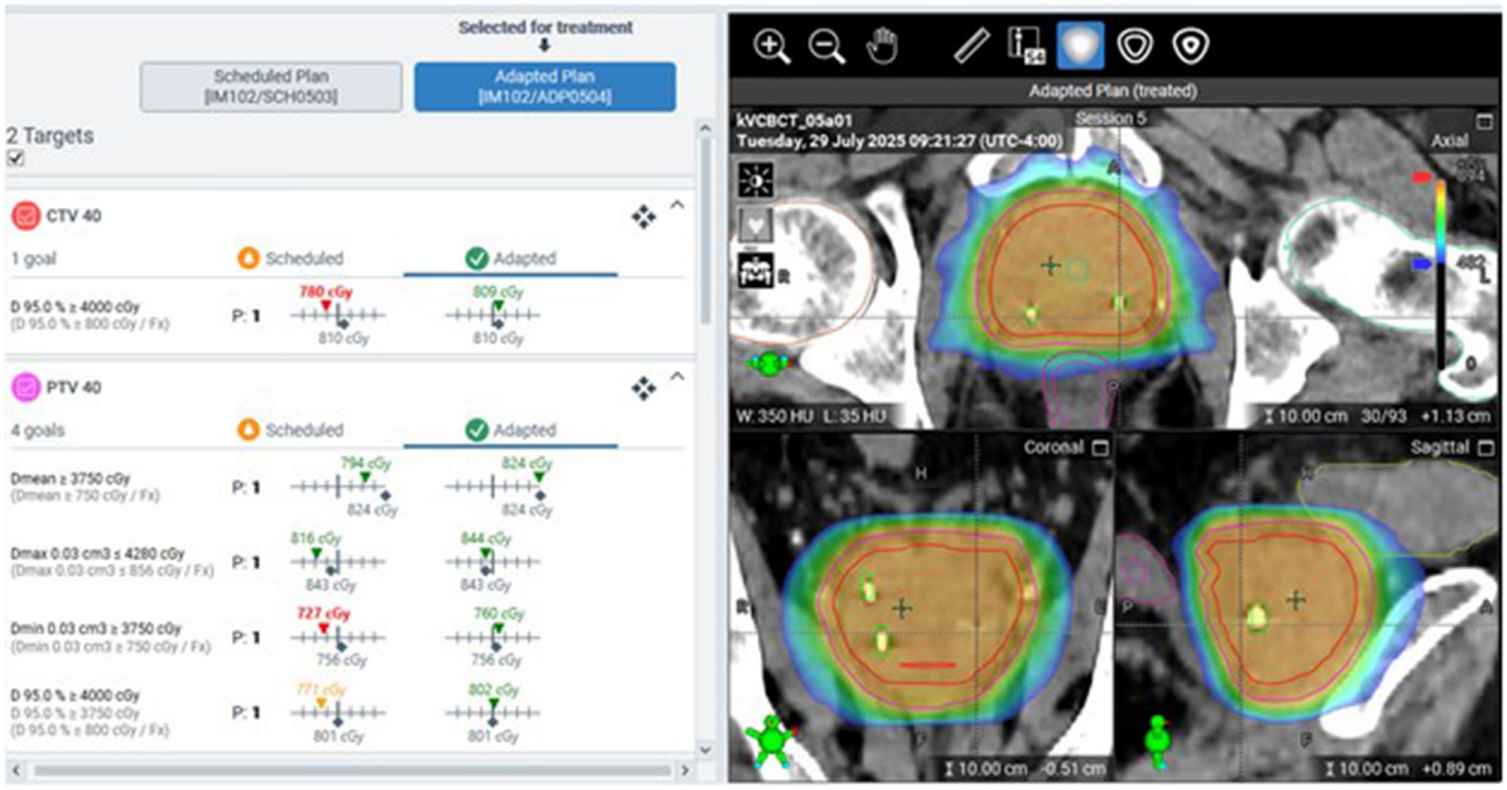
The adaptive plan is selected for treatment based on that day's CBCT. Fiducial markers are visible and used as the landmark for positioning verification during the on-couch adaptive process.
